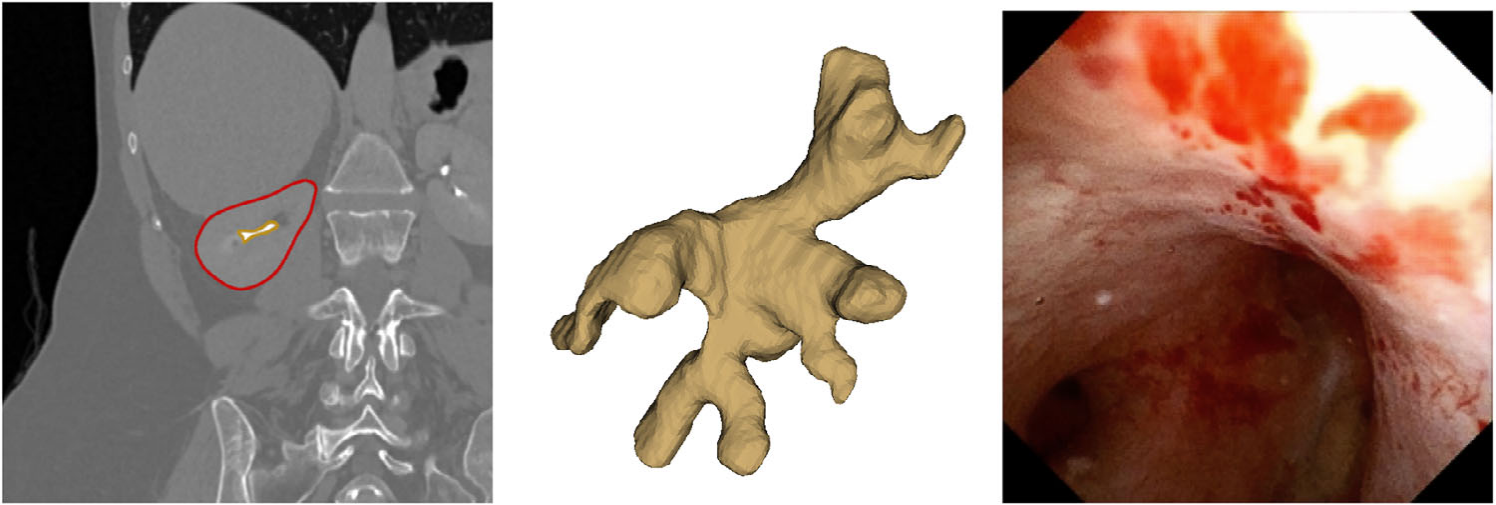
Kidney visualizations. (Left) Computerized Tomography (CT) imaging: Kidney outlined in red, renal pelvis outlined in yellow. (Middle) A rendered mesh of the renal pelvis. (Right) An actual ureteroscopy video. We note the vast differences between the preoperative and intraoperative modalities.
